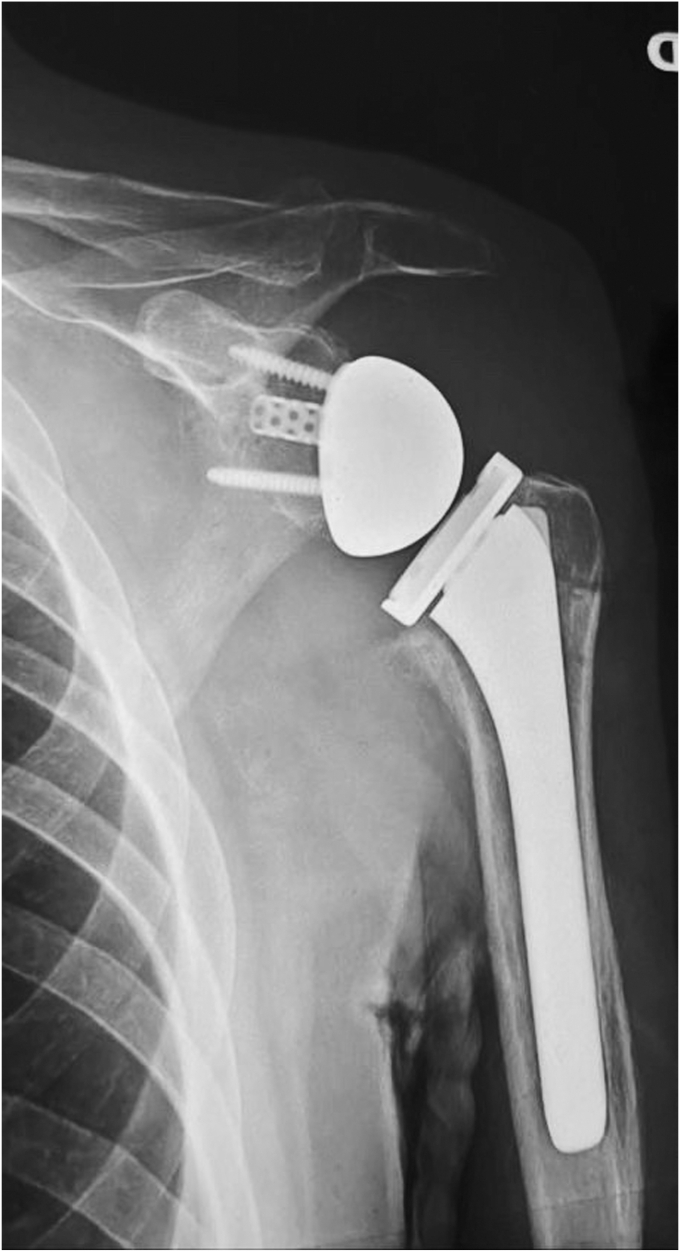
The correspondent postoperative X-Ray showing reduction of fracture site and alignment of the prosthesis with an uncemented stem.
